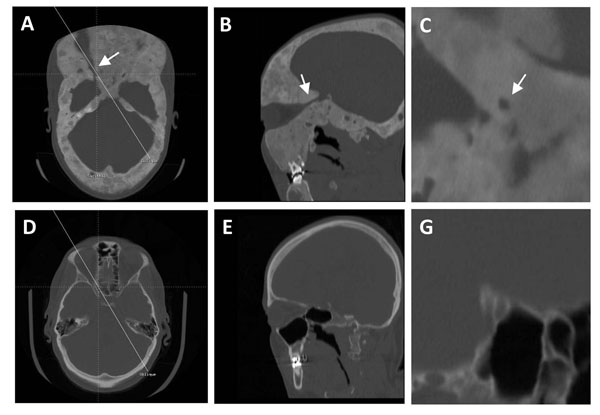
Fibrous dysplasia encasing the optic nerve compared to a normal optic canal. A-C) A patient with extensive fibrous dysplasia (FD). The arrow indicates the optic canal. D-G) CT of a normal and uninvolved optic canal. Several CT slices through the optic canal are shown: A&D) axial, B&E) oblique, and C&G) coronal. A case-control study by Lee et al [13] demonstrated that statistically significant narrowing of the optic canal by FD did not result in vision loss. Thus, observation with regular ophthalmologic examinations in patients with asymptomatic encasement was a reasonable treatment option and optic nerve decompression was not warranted. Adapted from reference [13]
